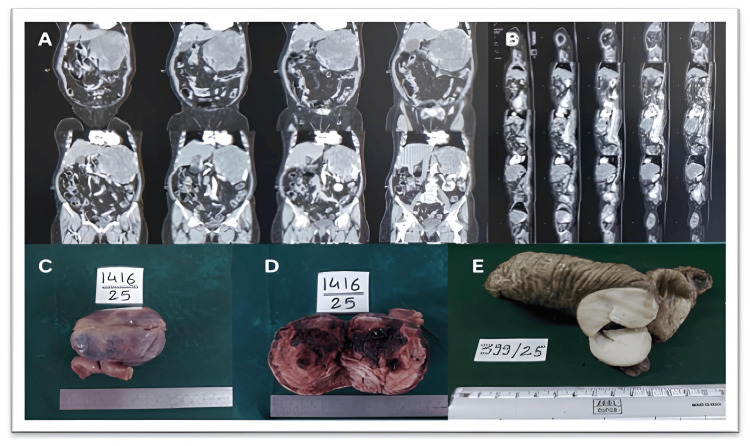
(A, B) Coronal and sagittal views of the CT scan of case 28 showing a large exophytic soft tissue mass epicentered in the stomach. Central areas of necrosis are seen with heterogeneous post-contrast enhancement. (C, D) Gross images of case 25 showing the ileum with a grayish-white mass. The cut section shows areas of hemorrhage and necrosis. (E) Gross image of case 12 showing the intestine with a solid whitish mass having smooth margins on the cut section.
